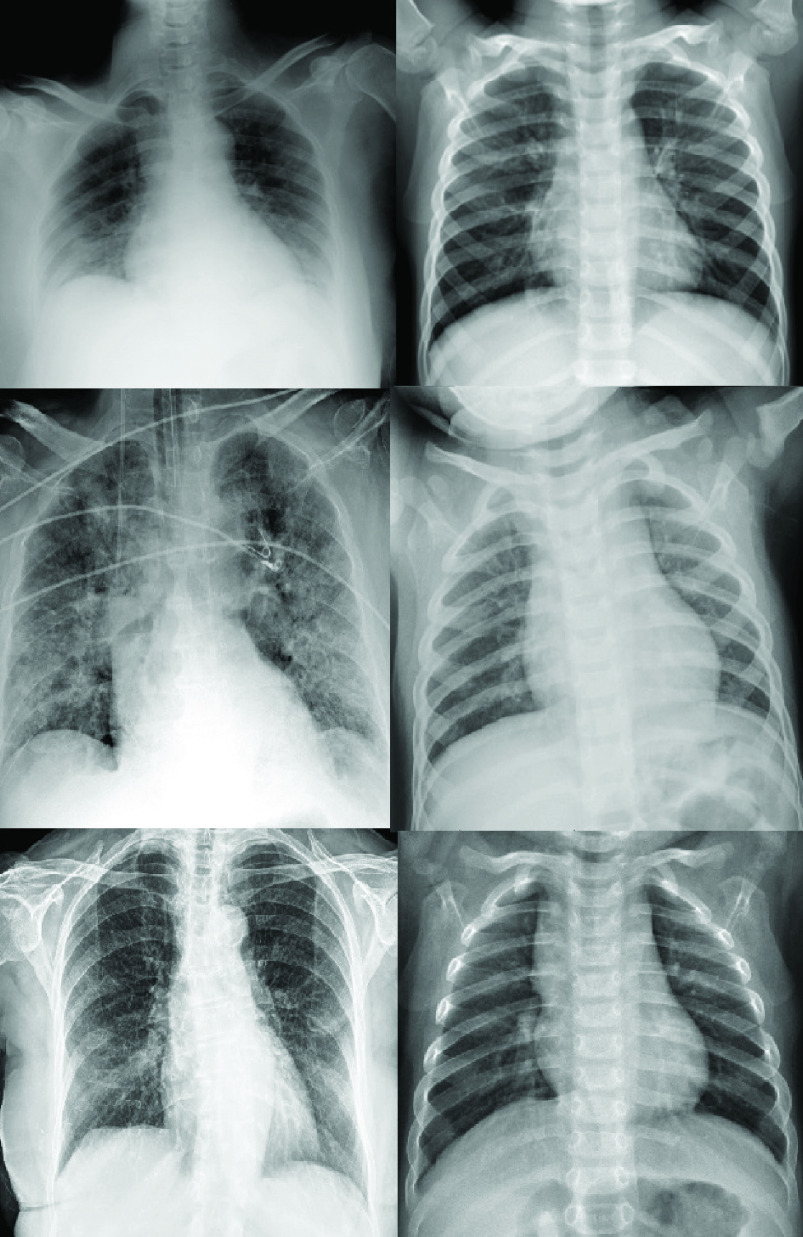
Left column, positive COVID-19 X-ray observations, right column, three negative COVID-19 observations. All of them were taken from the dataset used in this work.
